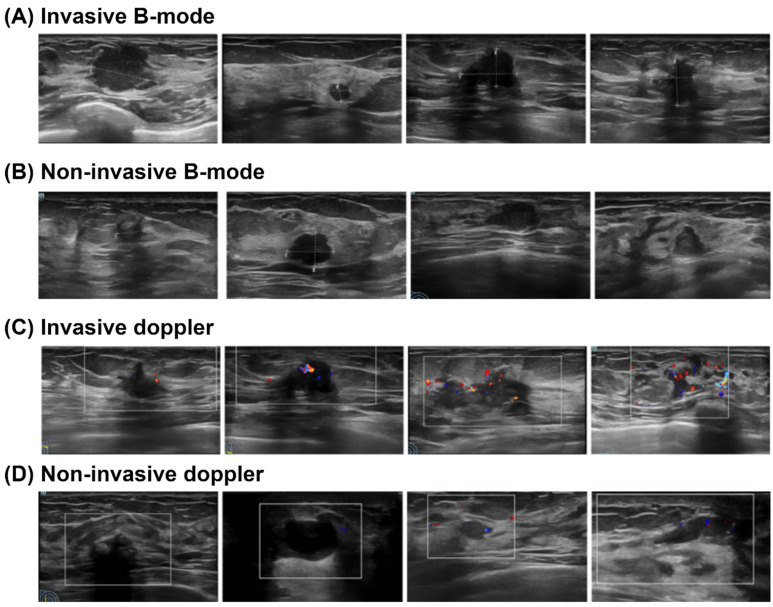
Representative ultrasound images of breast nodules with different pathological outcomes.
